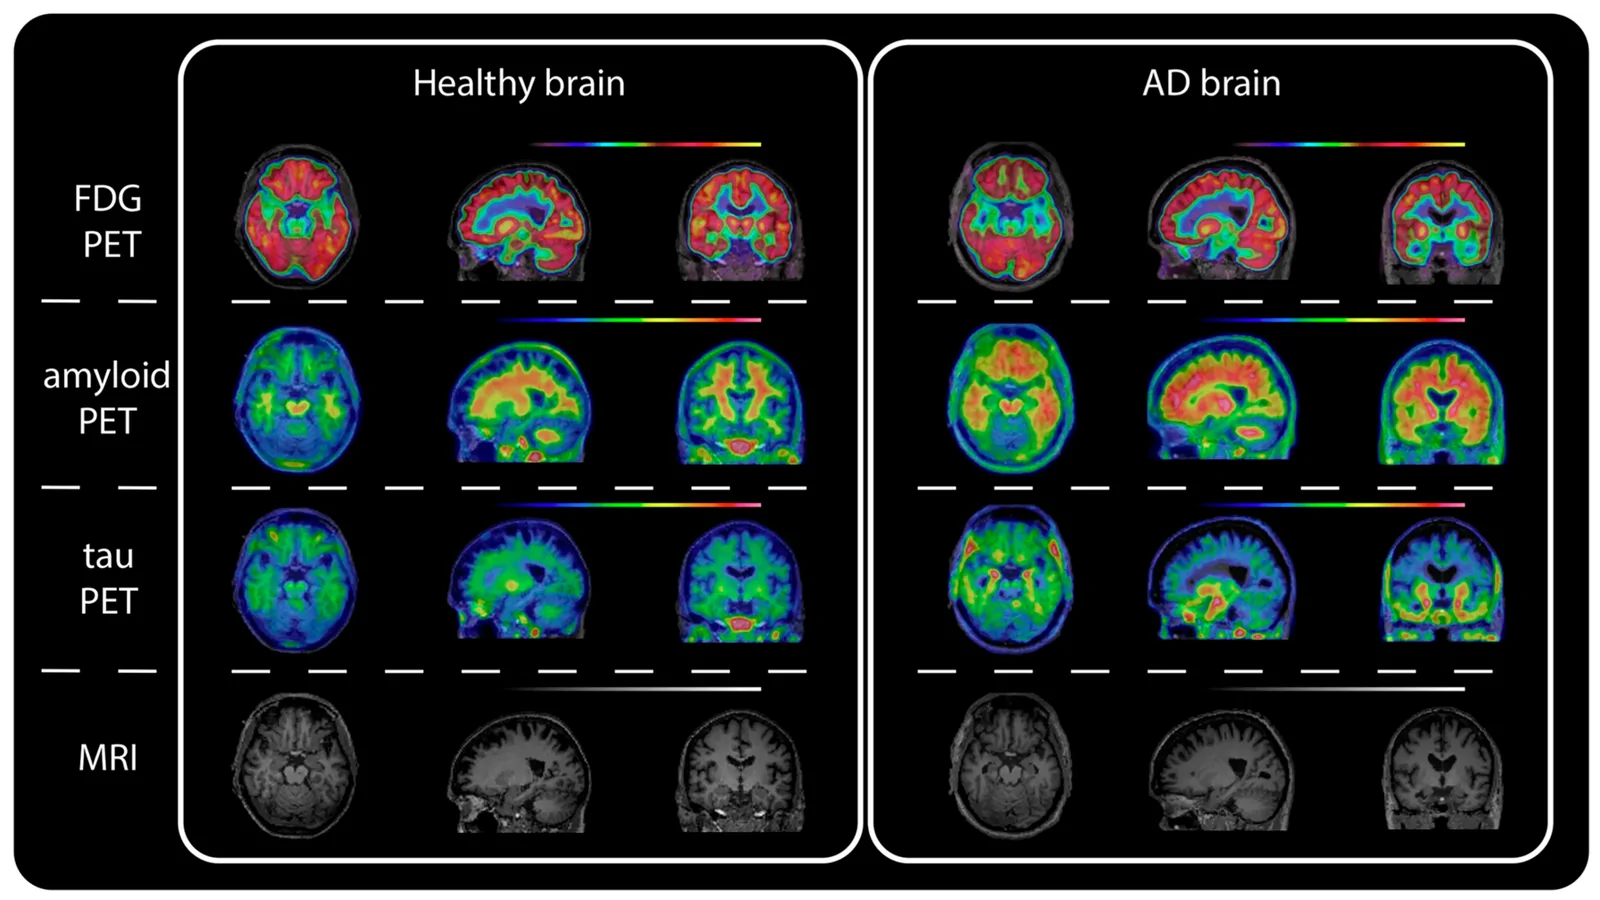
Multimodal neuroimaging features of healthy and AD brains. Representative FDG-PET, amyloid-PET, tau-PET, and sMRI images from the ADNI cohort. Compared with healthy controls, AD patients show reduced glucose metabolism, increased amyloid and tau deposition, and pronounced brain atrophy. Reproduced with permission from Ref. [18].
